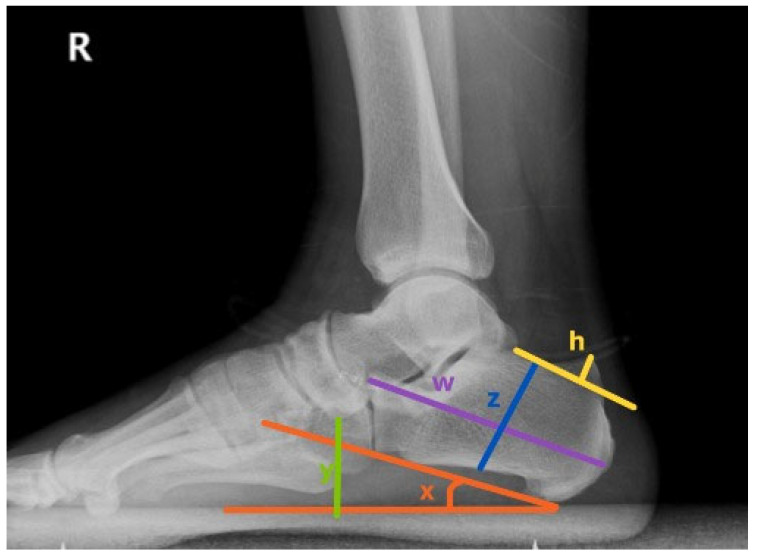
Radiographic measurement of foot. (h: Bump height, w: calcaneal length, x: calcaneal pitch angle, y: navicular height, z: calcaneal height).
